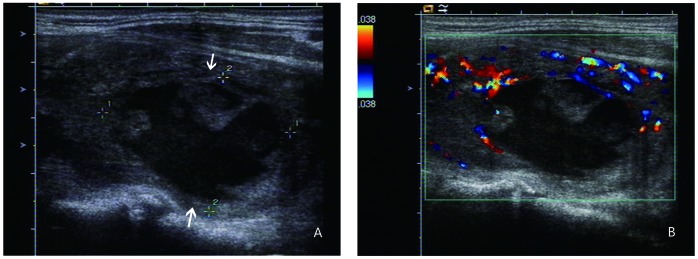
Thyroid tuberculosis in a 45-year-old patient. (A) Sonogram image revealing an ovoid-shaped, heterogeneous, hypoechoic nodule with ill-defined margins in the left thyroid lobe, resembling a cystic nodule with fluid space (white arrow). (B) Color Doppler examination revealing punctated and banded flow signals around the nodule.
